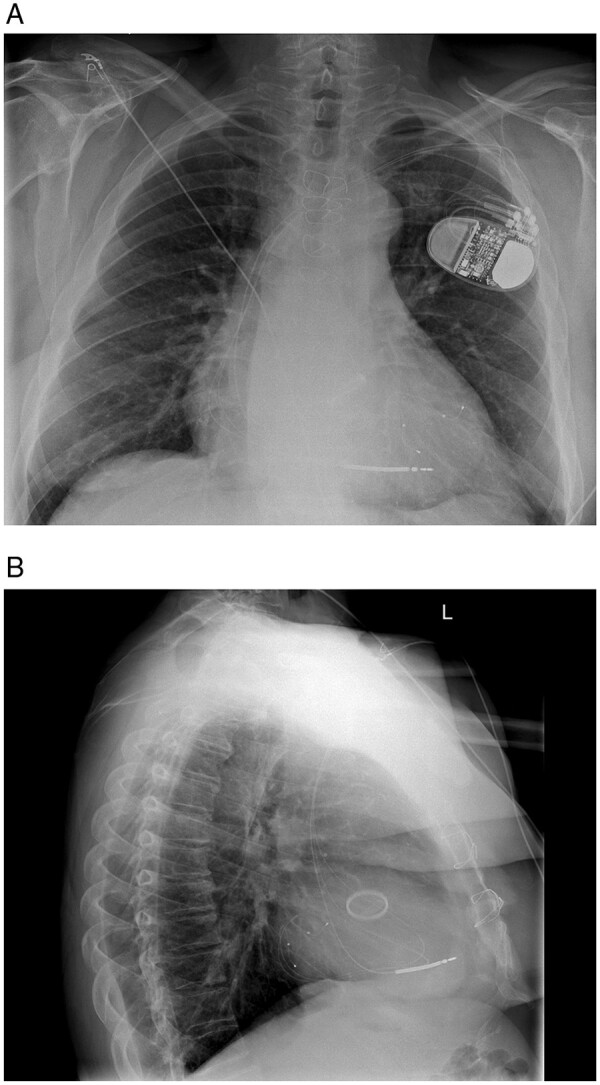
Representative posterior–anterior (*A*) and lateral (*B*) chest radiographs 1-day post-implantation showing a triventricular cardiac resynchronization therapy-defibrillator. Left ventricular leads are located in posterolateral and lateral coronary veins.
